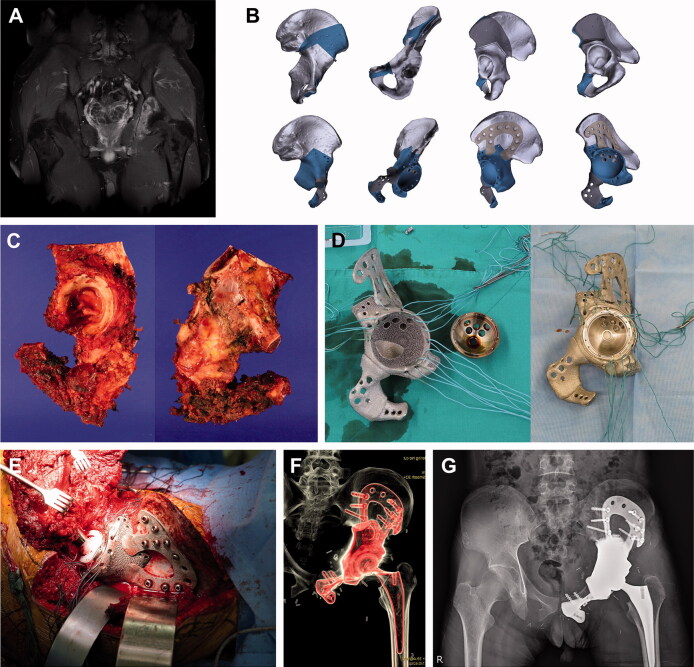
Pelvic reconstruction. Images of patient #5. (A) A preoperative gadolinium enhanced T1-weighted MR image showing Ewing sarcoma arising from the left acetabulum. (B) Graphical designs of the 3D-printed bone tumor resection guide (upper row) and implant (lower row). (C) Resected bone tumor as planned. Photographs of the (D) 3D-printed implant and THA cup before (left) and after conjugation (right). (E) Intraoperative photograph, (F) postoperative CT reconstruction image, and (G) plain radiograph showing pelvic reconstruction.
